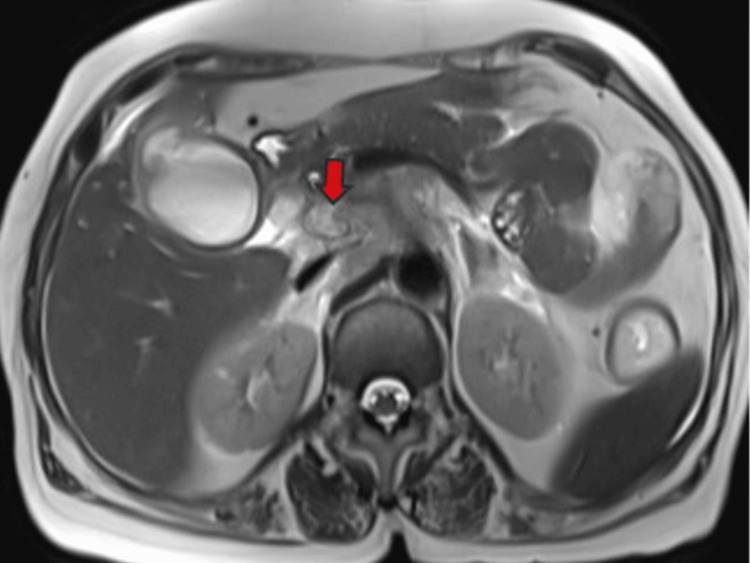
MR abdomen T2WI axial image showing a heterogeneous appearing enlarged necrotic pre-caval lymph node (red arrow). MR: Magnetic resonance; T2WI: T2-weighted imaging
